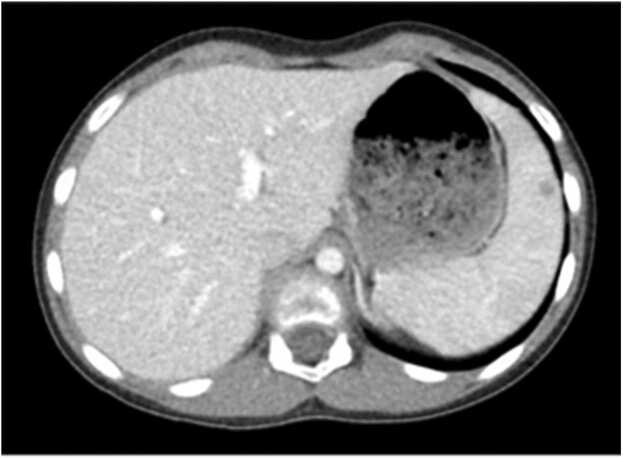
Case 7. CT thorax/abdomen with small nonspecific low-attenuation lesions in the spleen.
